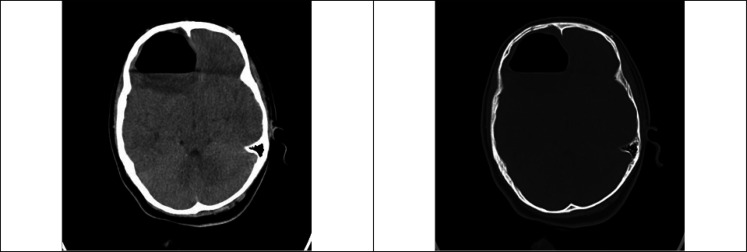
Spiral brain CT scan without contrast (axial cut) revealed air-fluid level in the frontal parenchyma with midline shift and compression effect on the anterior horn of the lateral ventricles in the right frontal lobe.
